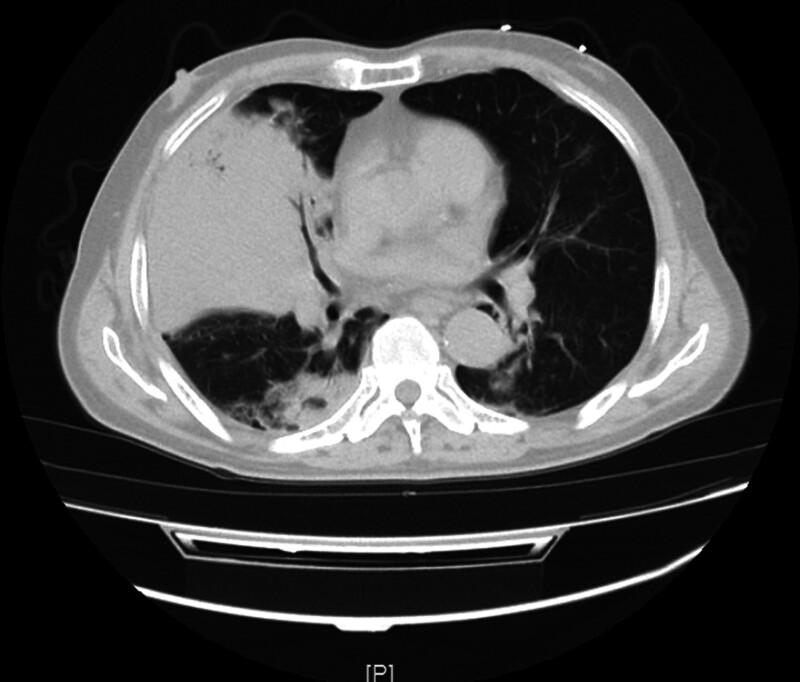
CT demonstrated inflammatory lesions in both lungs and consolidation of the right middle lobe.
